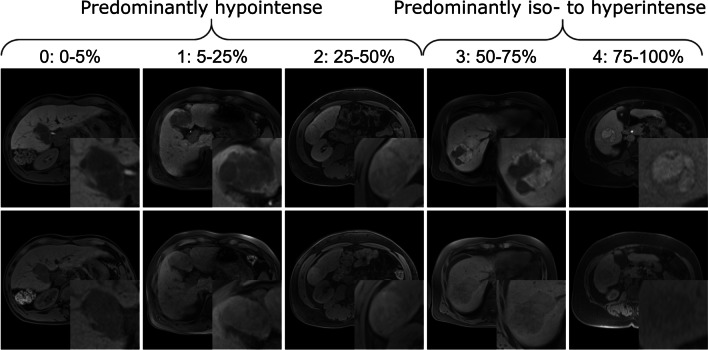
5-point scale for the subjective rating of Gd-EOB uptake by lesion area in the HBP. A lesion with a score of 3 or 4 is considered “predominantly iso- to hyperintense,” meaning that 50% or more of the total lesion area is iso- or hyperintense relative to the liver parenchyma in the HBP. Top row: HBP images; bottom row: precontrast T1-weighted images
